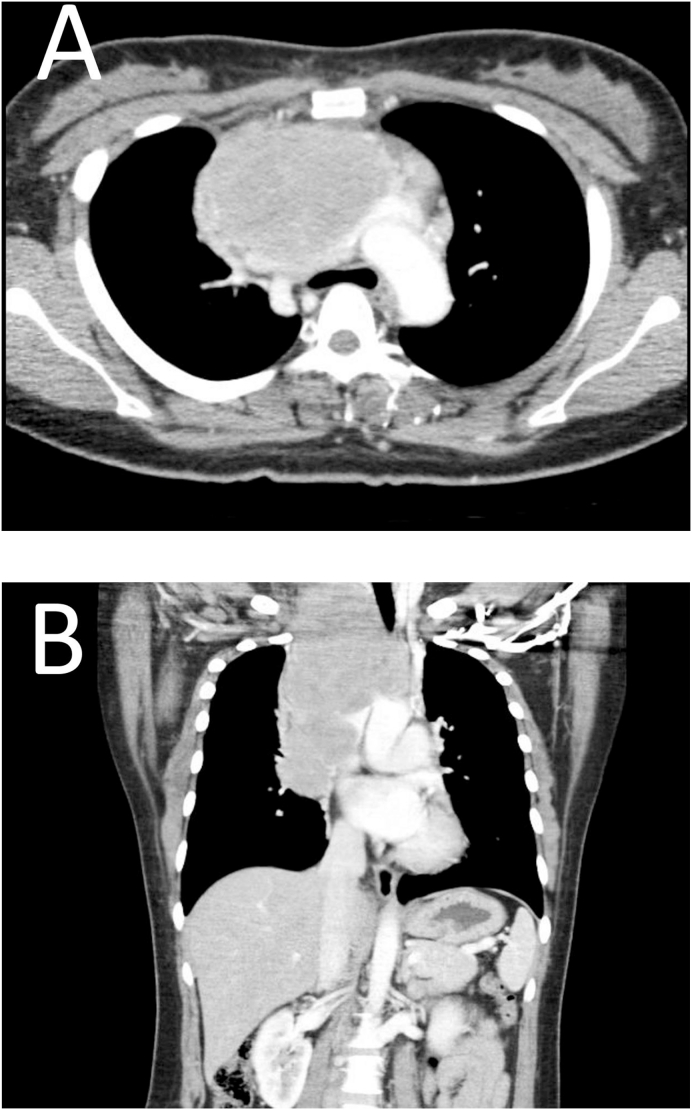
Chest CT showing a large mass at the anterior mediastinum in axial (A) and coronal (B) view.
